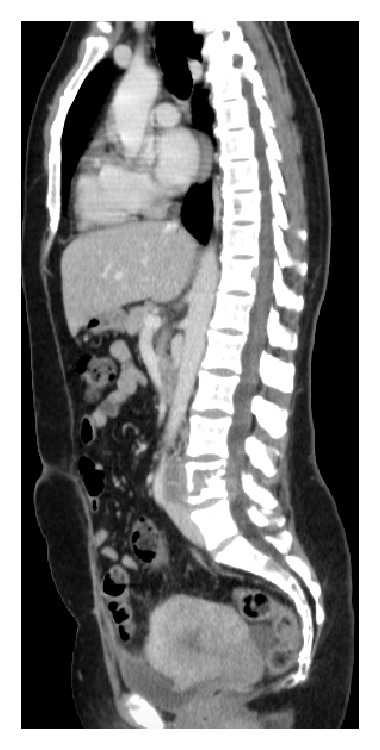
Contrast-enhanced CT. Sagittal view. There is a lytic lesion occupying the L4 vertebral body displaying a soft tissue component. The uterus is enlarged and depicts a nodular and lobulated contour, due to the presence of several leiomyomas.
